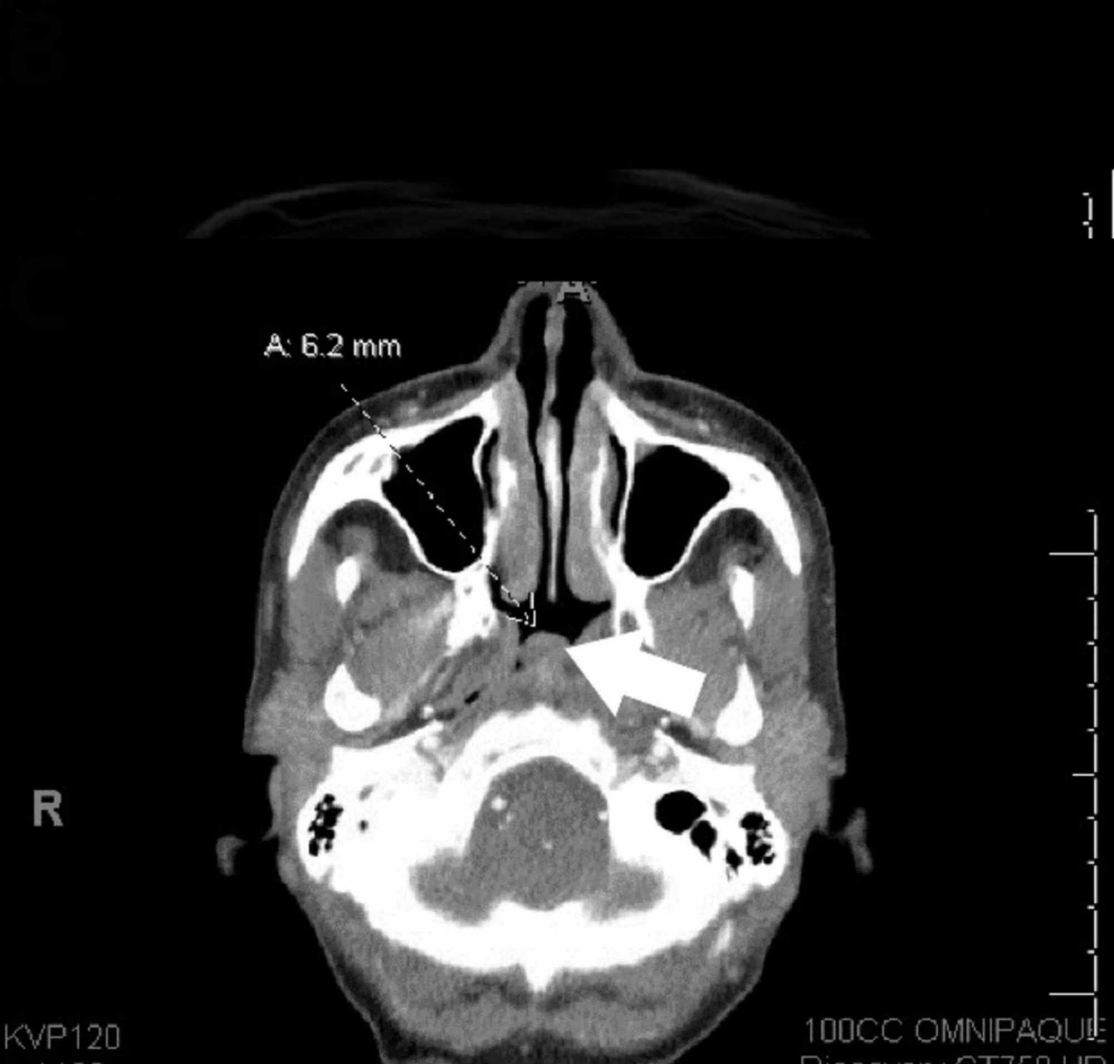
CT scans before treatment Heterogeneous cystic mass region in the nasopharynx (A) CT: computed tomography
